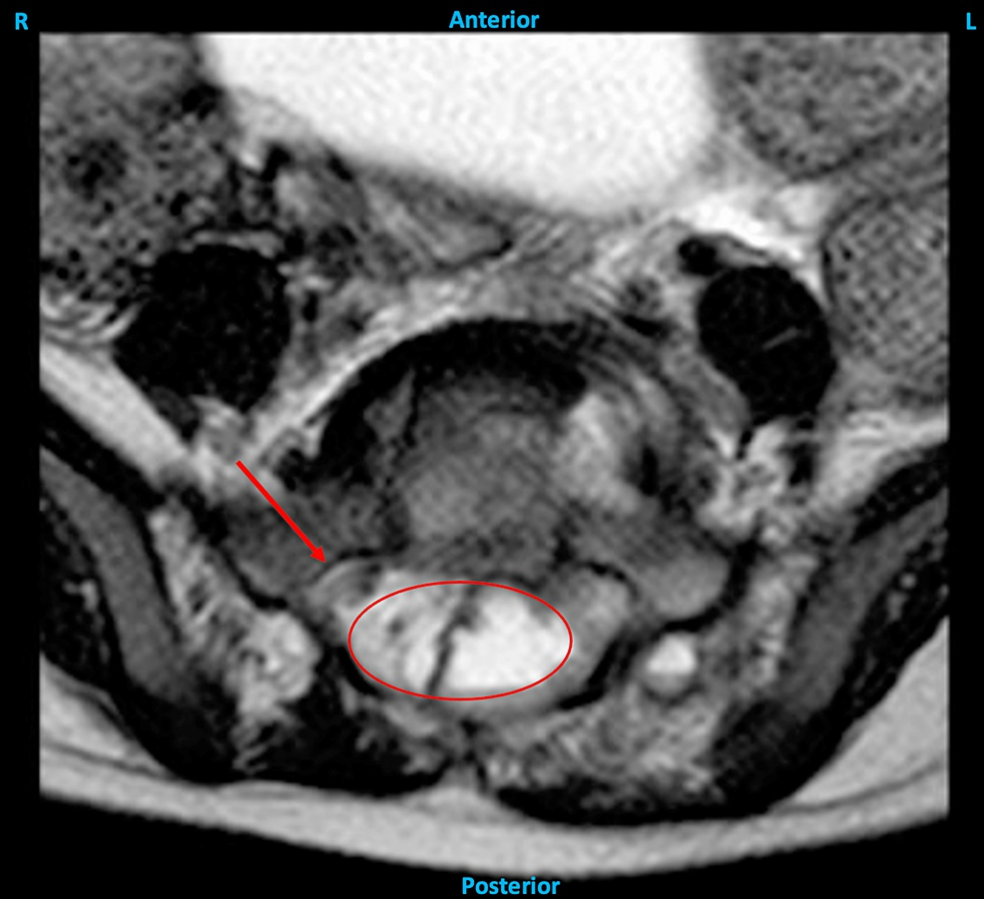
Axial MRI of the pelvis showing evidence of mass effect on the thecal sac (red circle) with rightward deviation of the sacral nerve roots (red arrow).
